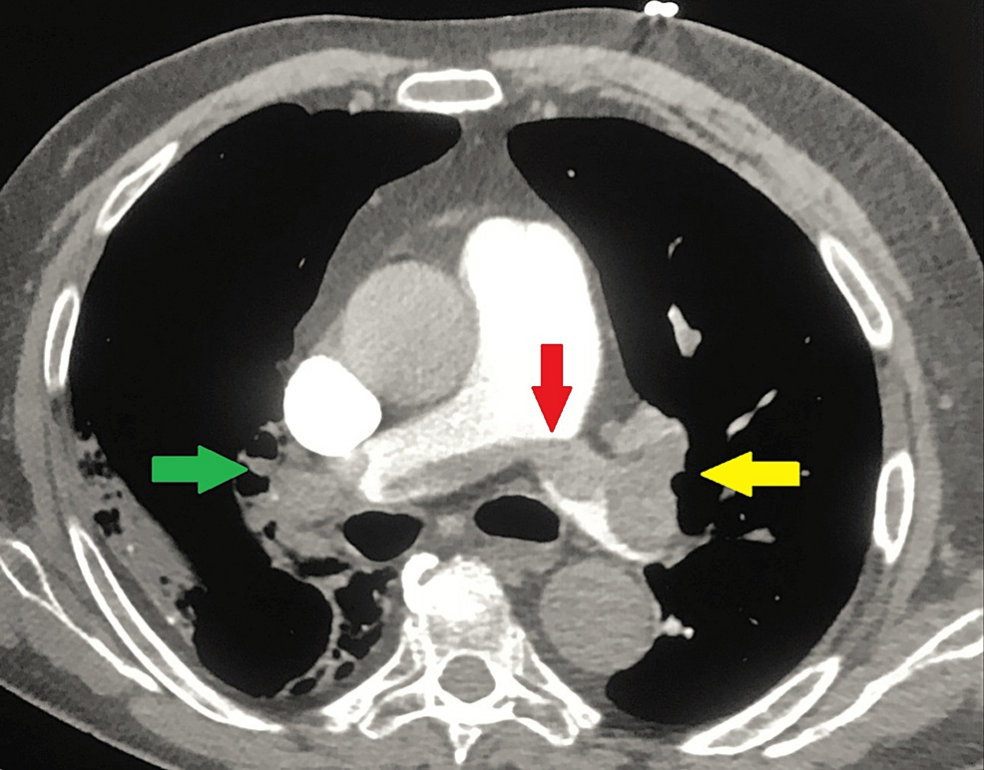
CT pulmonary angiography showing massive pulmonary embolism CT pulmonary angiography showing a red arrow pointing at massive pulmonary embolism communicating with both main pulmonary arteries, a yellow arrow pointing at the occlusion of left lobar arteries, and a green arrow pointing at the occlusion of right lobar arteries.
